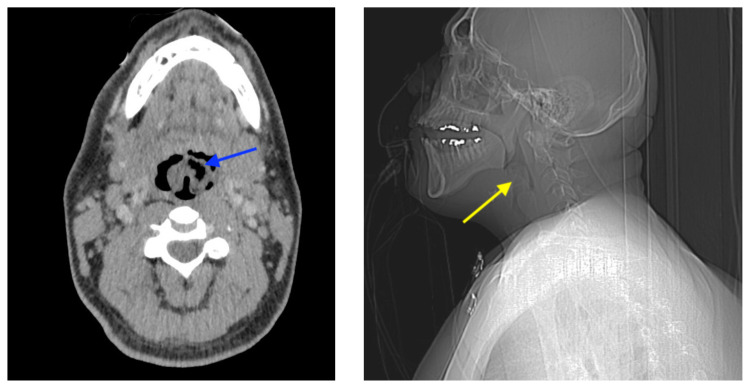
Sagittal CT Video Link: https://youtu.be/xUMQ2Kk8UeY
